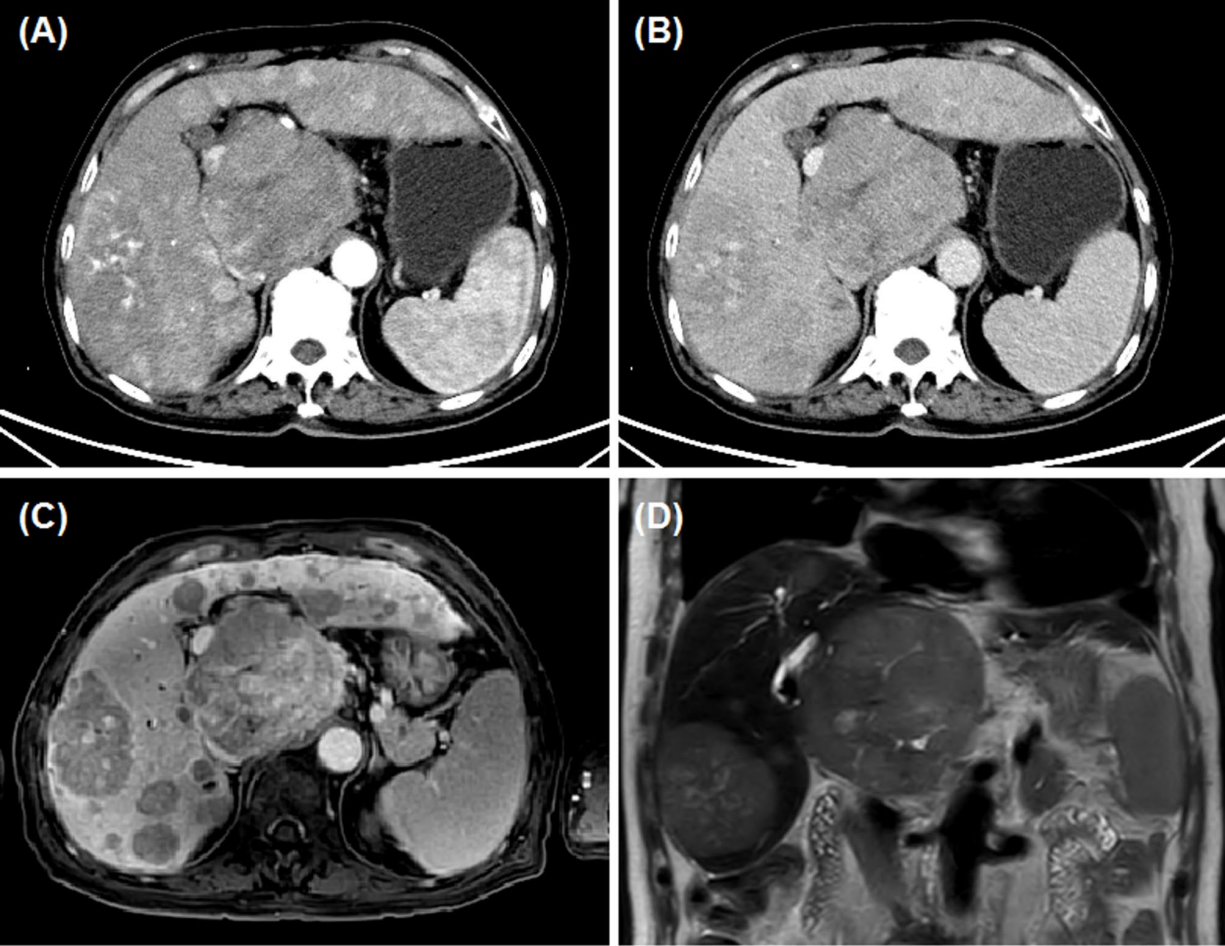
Abdominal contrast-enhanced CT and MRI for advanced primary HCC. (A-B) CT examination on admission revealed huge HCC with a maximum diameter of 94 mm, no portal vein invasion and multiple intrahepatic metastases. (C-D) MRI examination on admission revealed multiple cancer lesions in the left and right of liver lobes
